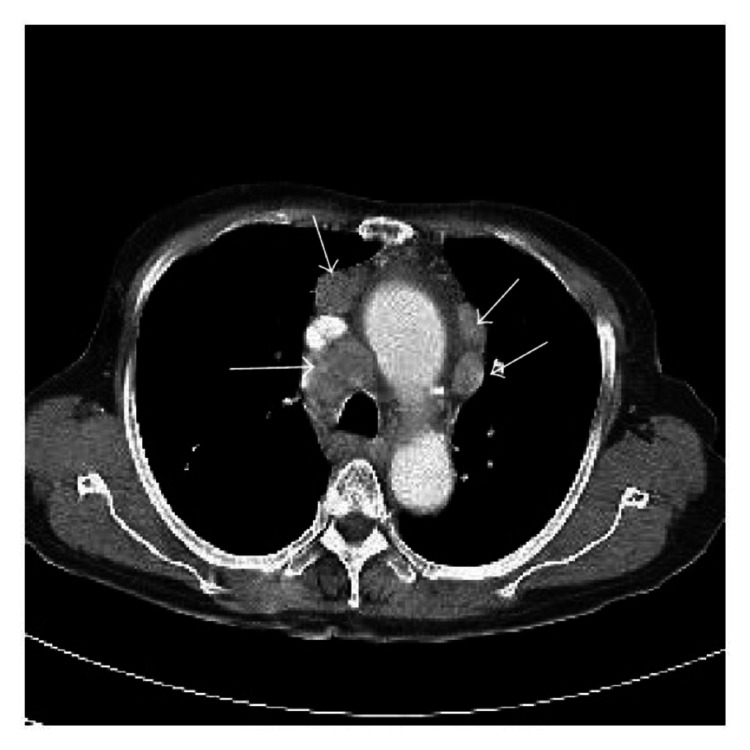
CT of the chest revealing mediastinal lymphadenopathy.
